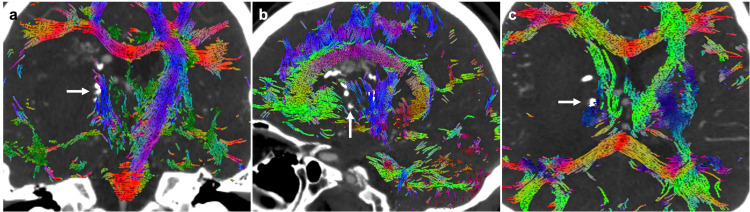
Post-embolization CTA and DTI fusion. Post-embolization CTA in the coronal (A), sagittal (B), and axial (C) slices with DTI tractography overlaid. The CST is shown to wrap posteromedial to the hemorrhage and embolized AVM feeders (arrow). CTA, computed tomography angiography; DTI, diffusion tensor imaging; CST, corticospinal tract; AVM, arteriovenous malformation.
